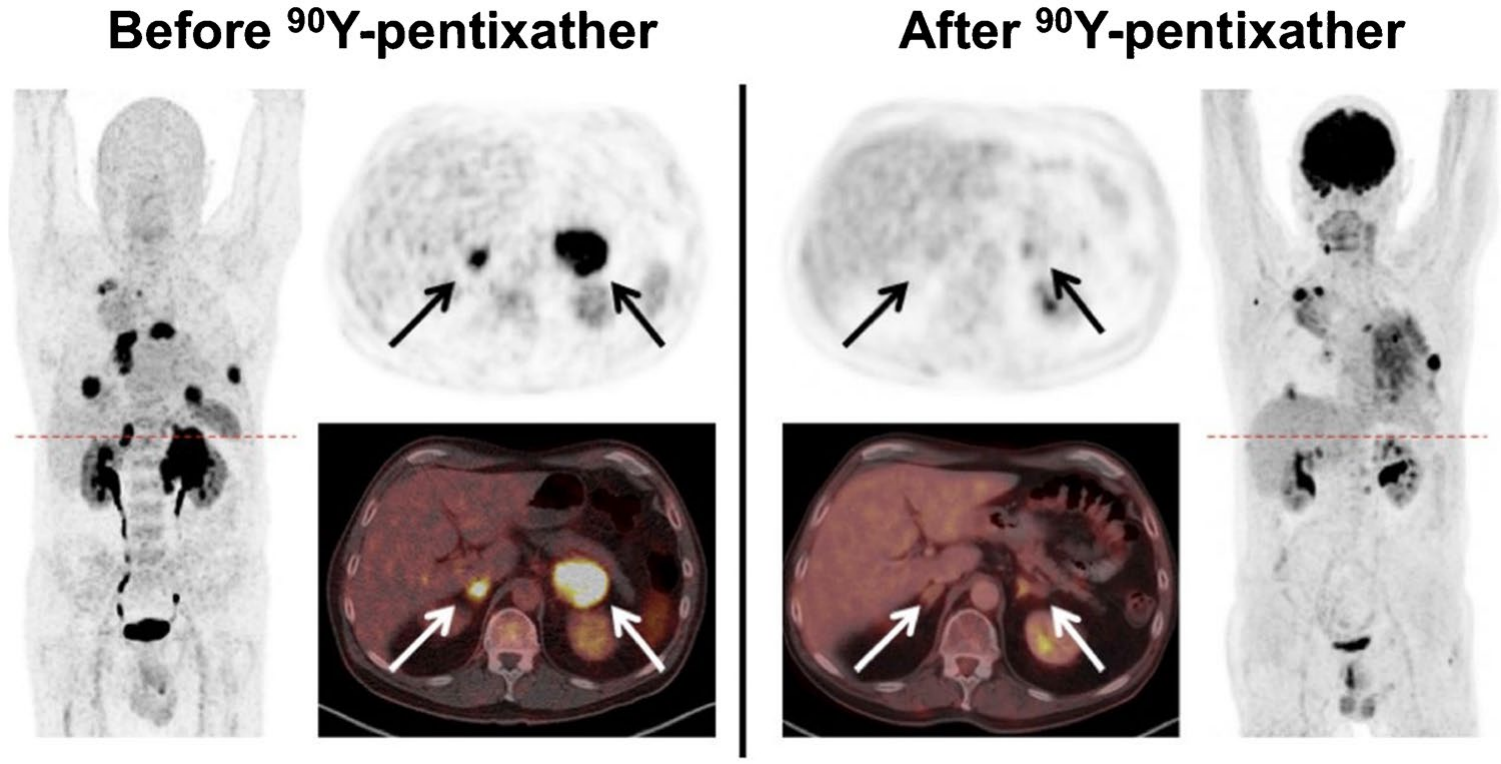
Partial response in a patient affected with diffuse large B cell lymphoma treated with CXCR4-targeted endoradiotherapy and additional radioimmunotherapy. Pretherapeutic [^68^Ga]PentixaFor (left) and posttherapeutic [^18^F]FDG PET/CT (right) after tandem treatment using [^90^Y]PentixaTher and [^90^Y]Ibritumomab-Tiuxetan (Zevalin). Posttherapy scans demonstrated reduction of lesions in the kidneys, adrenals (arrows), lung, and nodal disease manifestations. Note that diffuse radiotracer accumulation in the lung on [^18^F]FDG maximum intensity projection on the right was due to pneumonia. Modified from Lapa et al., Journal of Nuclear Medicine Jan 2019, 60 (1) 60–64 [15], © by the Society of Nuclear Medicine and Molecular Imaging, Inc
